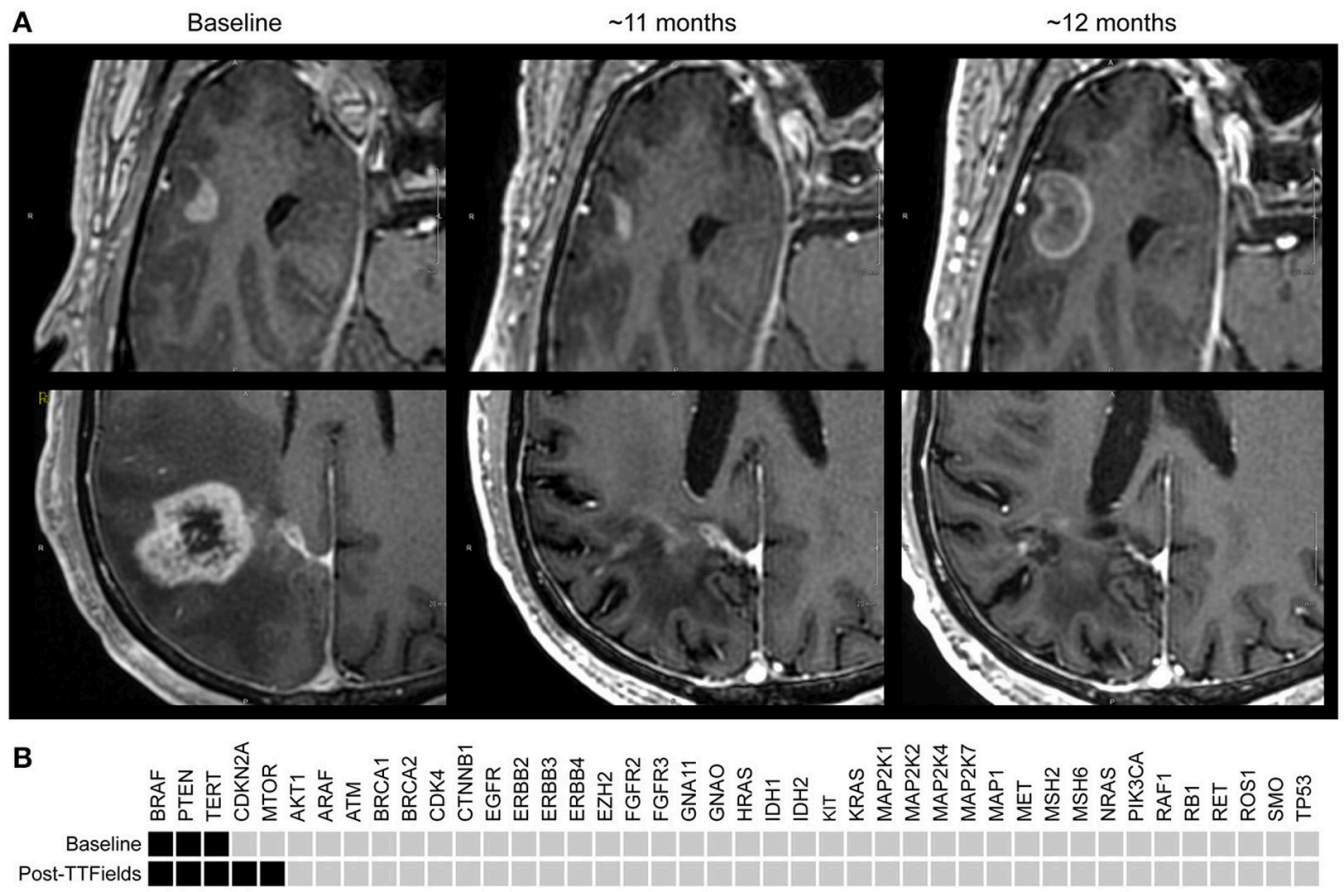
(A) T1+contrast MRI images: Upper panels are right temporal lobe; lower panels are corresponding right parietal images. *Baseline* (Aug. 2016) demonstrates the first appearance a temporal lobe lesion ~2 months post radiation/temozolomide; the lower panel demonstrates the primary GB. Middle section ~*11 months* later (June 2017) demonstrates slightly less enhancement of the temporal lobe lesion, and a dramatic reduction in enhancement and size of the parietal lobe lesion with decreased edema and treatment related cerebral atrophy. At ~*12 months* (Aug. 2017) the temporal lobe lesion has increased to 18 × 13 mm; the parietal lobe remains stable and in remission. (B) StrataNGS cancer hotspot sequencing was performed on the resection of the primary parietal lobe lesion, which possessed mutations in *BRAF* (V600E), *PTEN* (319fs), and the *TERT* promoter (C228T). Following progression on TTFields, the separate anterior temporal lesion was resected and demonstrated *BRAF, PTEN*, and *TERT* alterations, and the acquisition of a deep deletion of *CDK2NA* and an activating mutation in *mTOR* (V2006I). No other pathologic alterations were identified in the remaining 47 genes of the 88 genes assessed.
